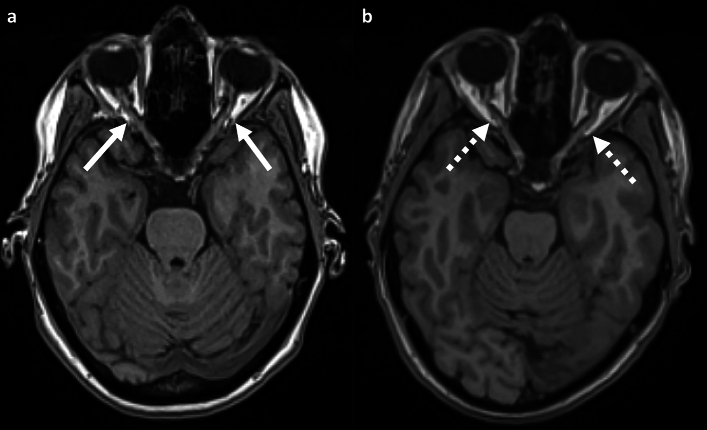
Variations in the course of the optic nerve on axial T1-weighted images; (a) 37-year-old female participant with straight course of the optic nerve (white arrow). (b) 39-year-old female participant with meandering course within the orbit (dotted white arrow).
